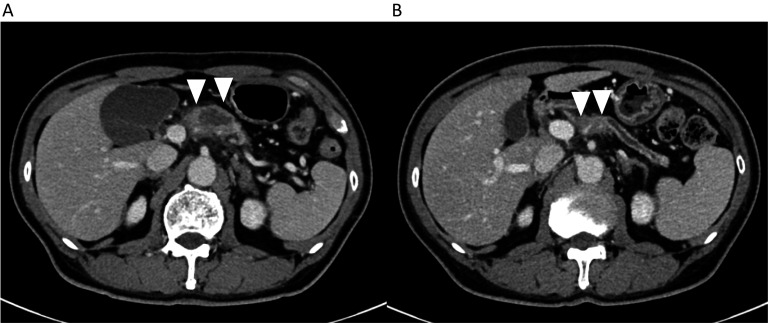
A Contrast-enhanced computed tomography shows the tumor before chemotherapy. B Contrast-enhanced computed tomography shows the tumor shrink from 3 to 2 cm after chemotherapy
